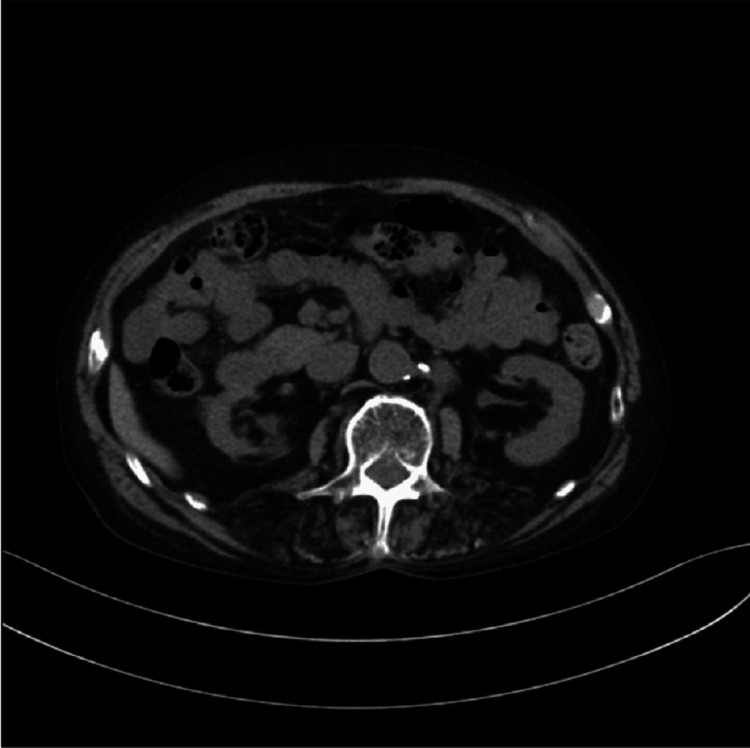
Metastatic sites in patient 5 Computed tomography imaging revealed no apparent visceral metastases.
